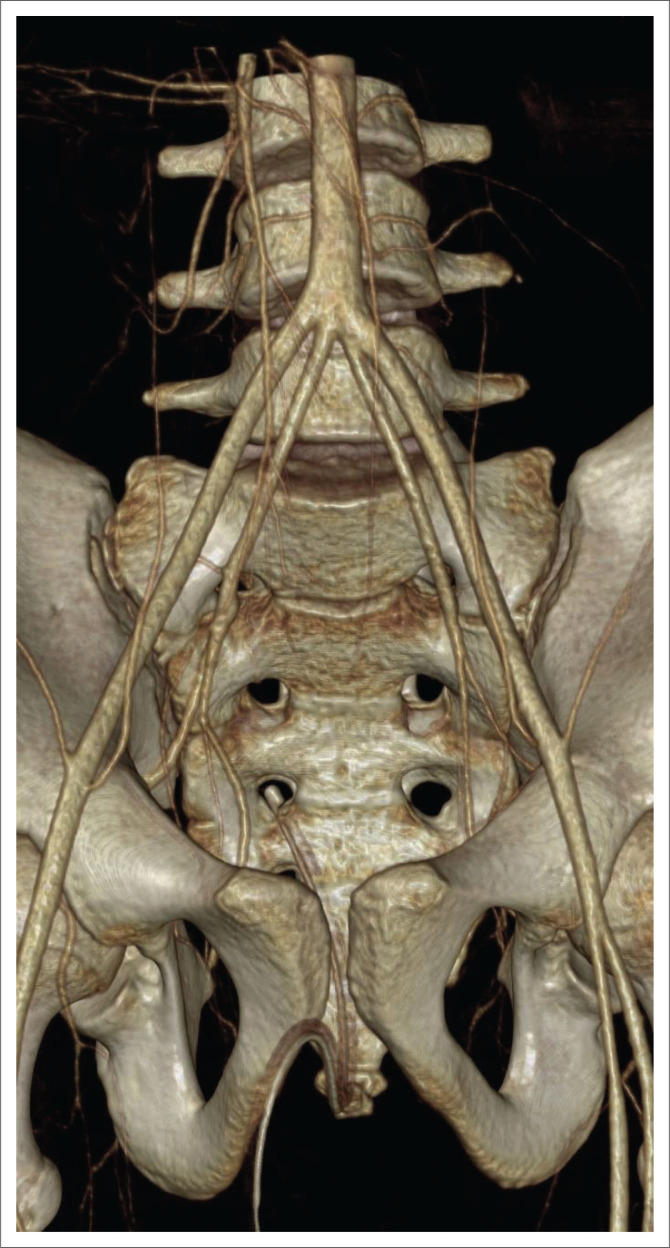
A 3D computed tomography angiogram of the pelvis depicting quadrifurcation of the terminal aorta.
